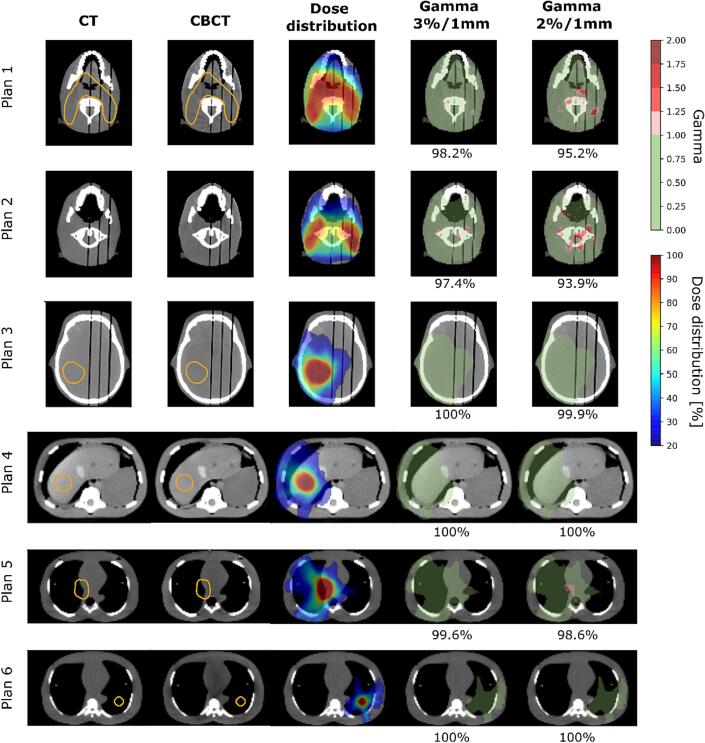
Examples of CT and CBCT images as well as corresponding planned dose distribution on the CT image and gamma maps for all investigated plans. Orange contours show the target, if present on the given slice. The target volumes for Plan 1 and Plan 2 were the same. The slice positions were chosen based on the location of the largest dose difference. Green shading on the gamma maps indicates dose regions where gamma < 1. The gamma maps show both over- and under-dosage with a red color. Dose distribution is shown with 20 % isodose as lower threshold.
